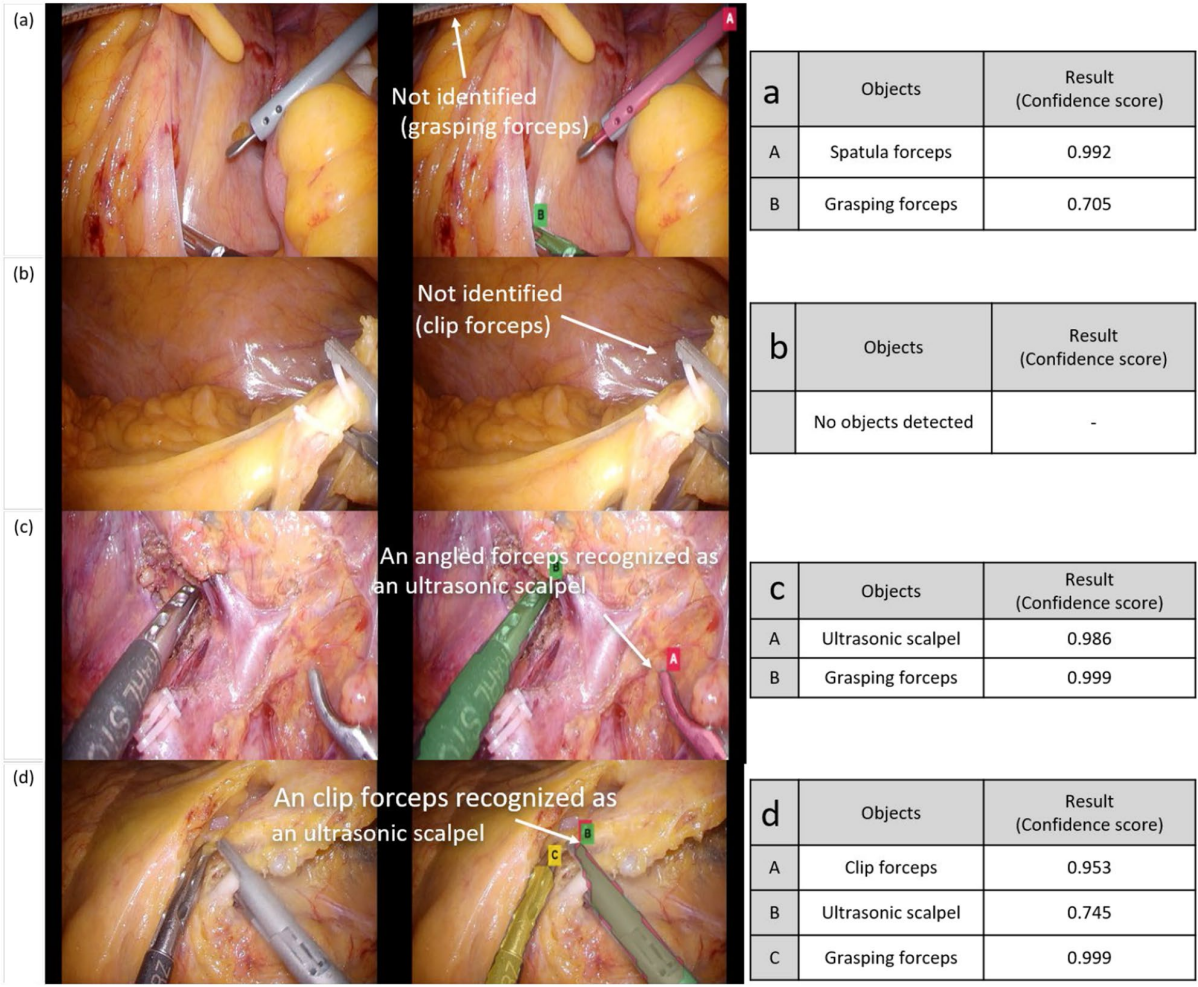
Representative images demonstrating inaccurate results. The images on the right side are original. The images in the middle are test results. The images on the right show the confidence scores of each result. (a) A grasping forceps and 1 spatula forceps were detected accurately, but 1 of the 2 grasping forceps in the image was not detected correctly; (b) the clip forceps was not identified correctly; (c) the angled forceps was recognized as an ultrasonic scalpel incorrectly; and (d) the clip forceps was identified correctly but was also recognized as an ultrasonic scalpel.
